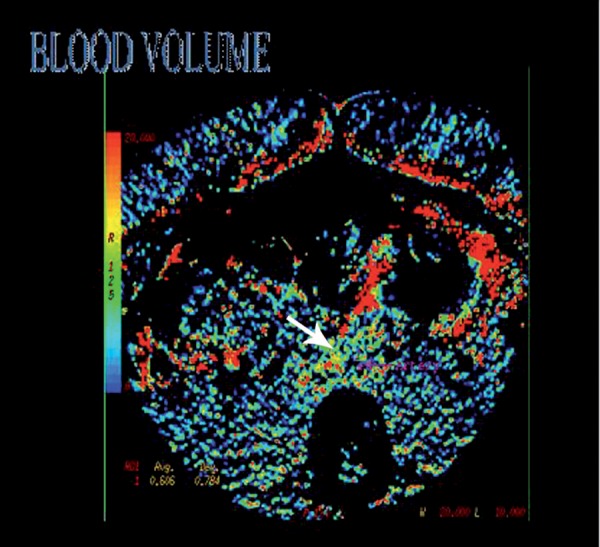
Perfusion CT scan with MDCT 64 shows the retroperitoneal mass (arrow) without blood flow signals.
